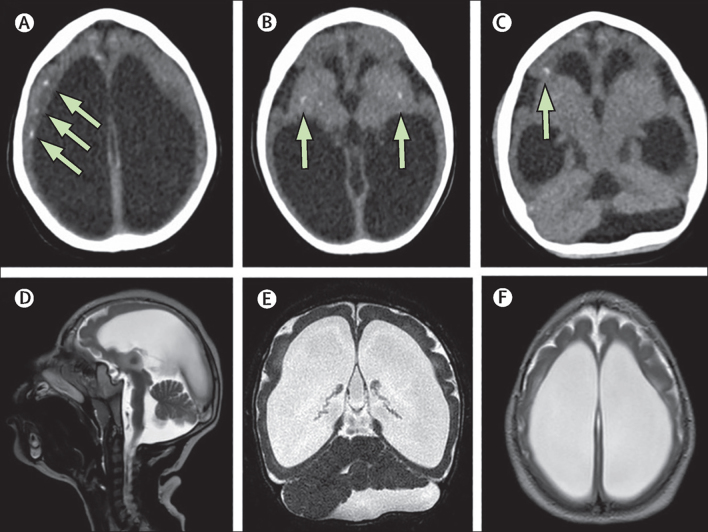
Brain CT and MRI scans of an Angolan child with microcephaly (A) Compensatory ventriculomegaly and calcification areas in the subcortical region are shown by green arrows. In (B) and (C), calcification in the basal ganglia is shown by the green arrows. (D) Brainstem hypoplasia. (E) Dysgenesis of the cerebellum. (F) Pachygyria.
